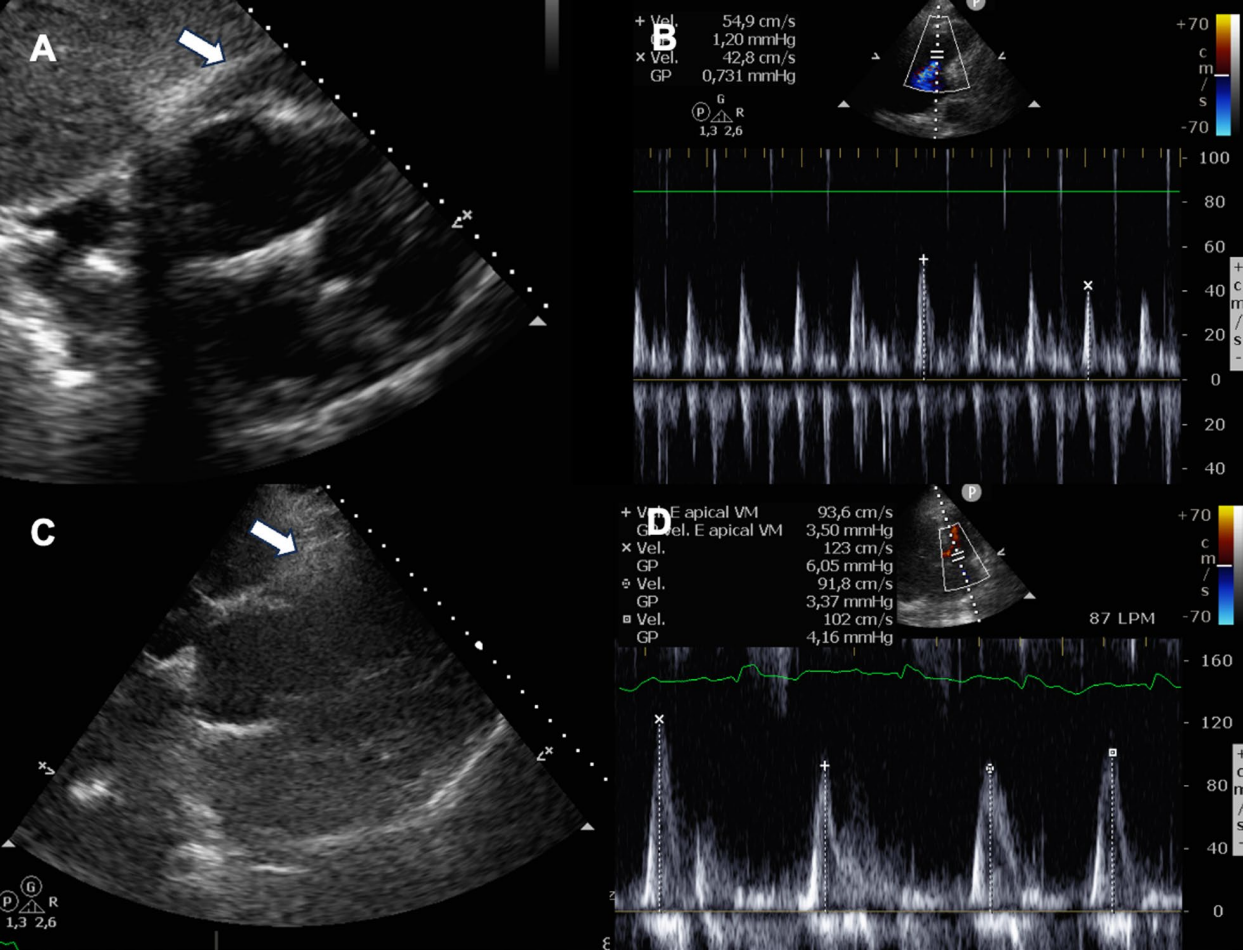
A 2D Echocardiogram: Subxiphoid view showing pericardial fluid with right ventricular collapse consistent with cardiac tamponade (arrow); B Doppler echocardiography: variation in mitral peak E velocity more than 25–40% with inspiration; C 2D Echocardiogram: Subxiphoid view showing no pericardial effusion (arrow); D Doppler echocardiography: mitral peak velocity without abnormal variation
